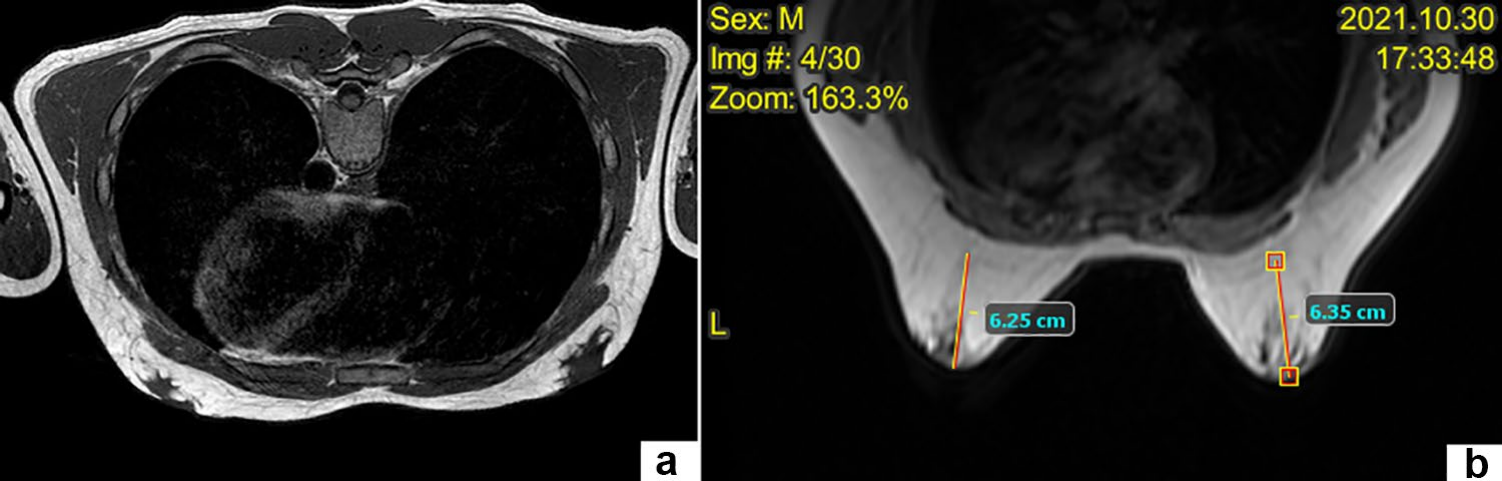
MRI was helpful to differentiate gynecomastia (a) from adipomastia (b)
